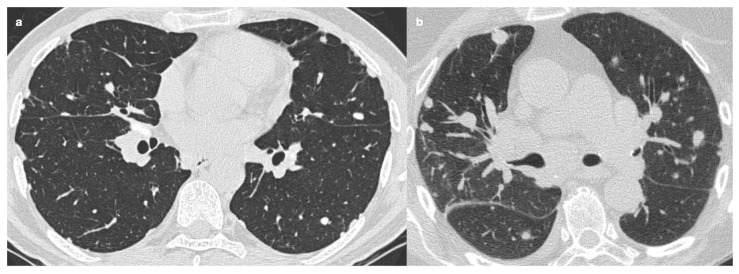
The HRCT scan of a patient with known sarcoidosis shows the presence of diffuse bilateral nodules (a). In a patient with known primary malignancy, the lung CT scan shows the presence of metastatic nodules in both lungs of heterogeneous sizes (b).
